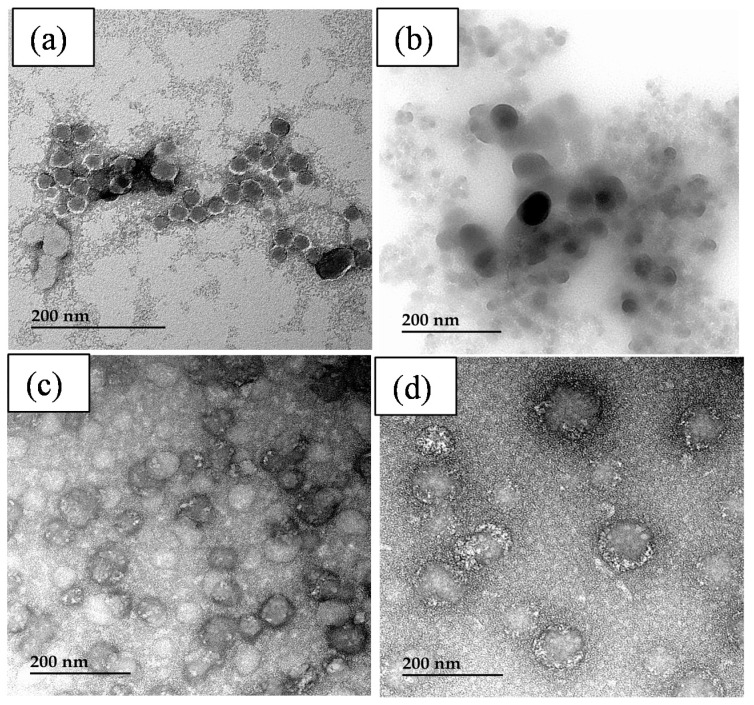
TEM images of starch particles stemming from hydrolysates of starch modified by supercritical CO_2_ (a); ultrasonication (b); HEBM under dry (c) and wet (d) conditions.
